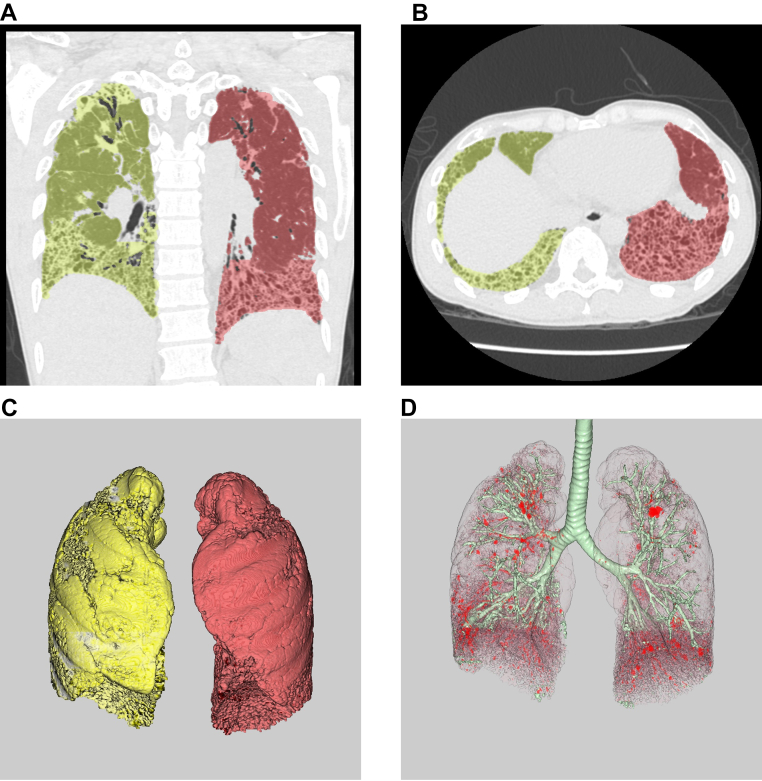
Analysis of quantitative normal lung volume on chest high-resolution CT (HRCT) imaging using a 3-dimensional image analysis system (Synapse Vincent version 6.1; Fujifilm Corporation). A-C, The lung areas on HRCT imaging are identified—coronal section (A) and axial section (B)—and the total lung volume is abstracted and synthesized (C). D, The red spaces in the lungs represent abnormal areas identified by –1024 to –950 Hounsfield units (HU), and the areas of –950 to –600 HU are defined as normal lung volume.
